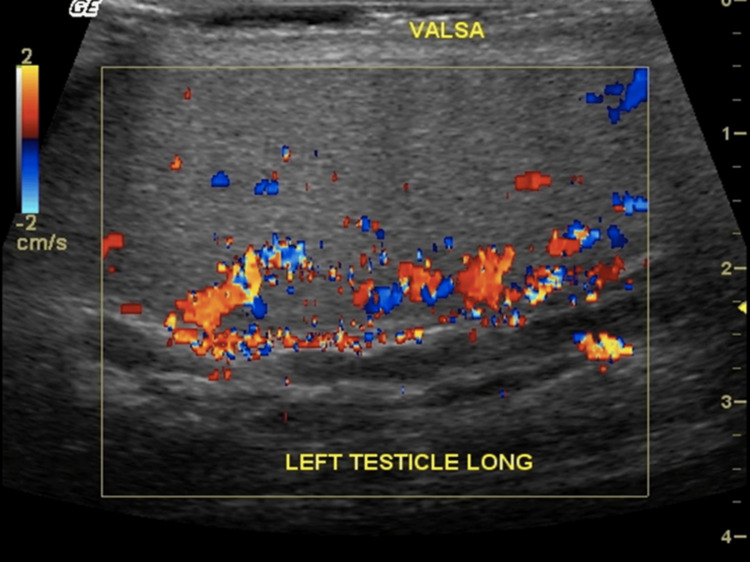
Absent vascular flow within a thrombosed varicocele shown on color Doppler US.
